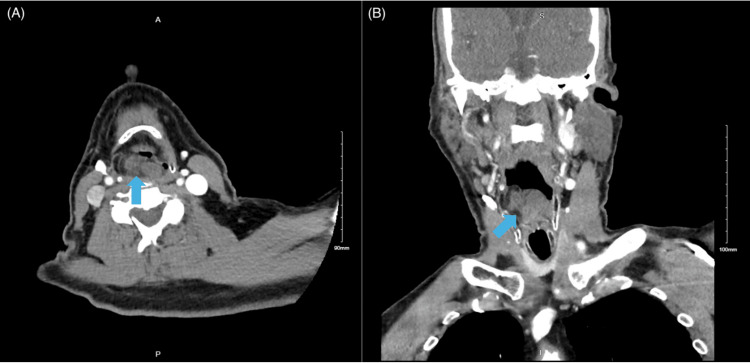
Preoperative axial (A) and coronal (B) CT soft tissue of the neck with contrast showing an 1.8x2.8x2.0 cm right-sided supraglottic soft tissue mass (blue arrows) partially obstructing the larynx.
